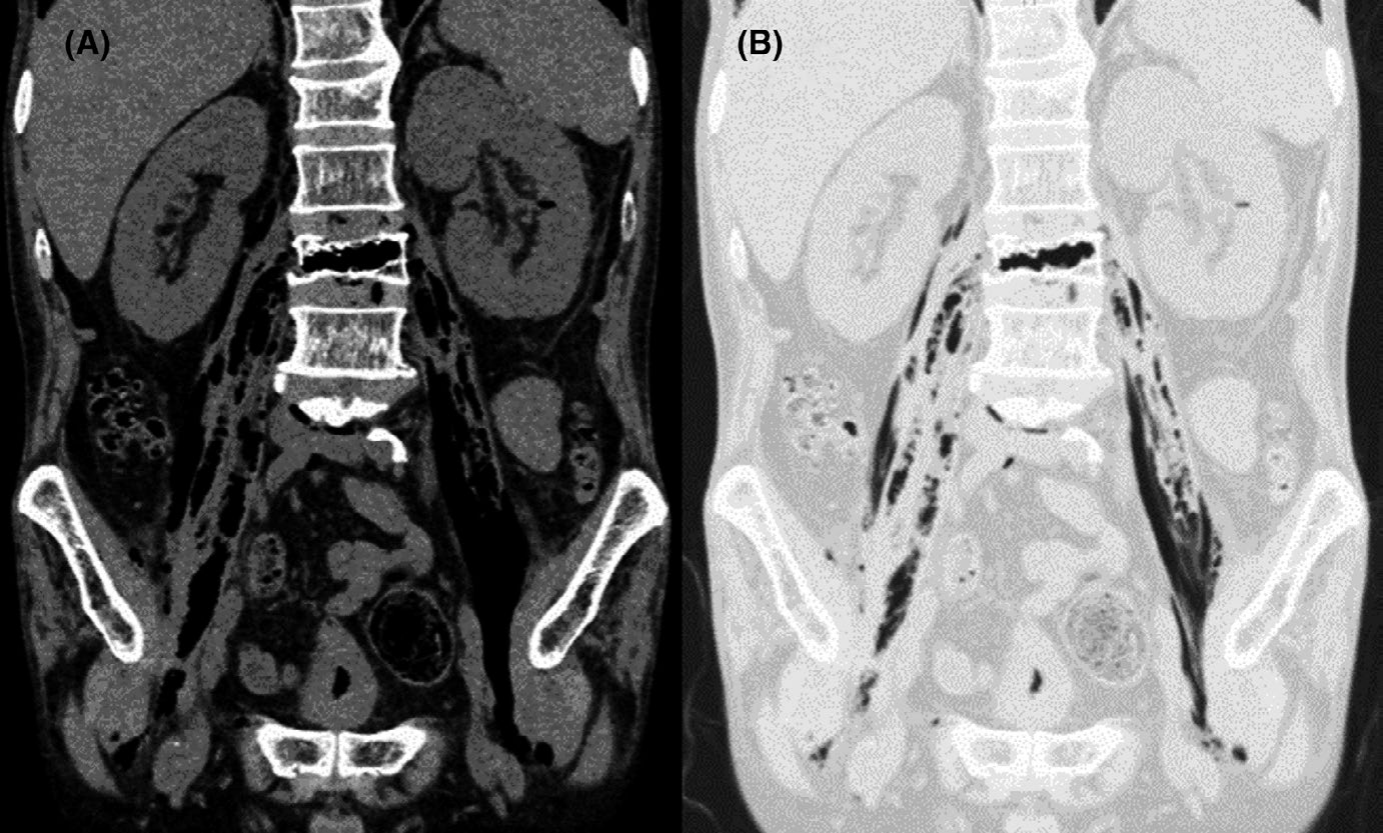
Coronal view on abdominopelvic computed tomography on admission, showing gas widely distributed across the left renal parenchyma, bilateral iliopsoas, and third lumbar vertebra. (A) Standard window setting. (B) Pulmonary window setting
